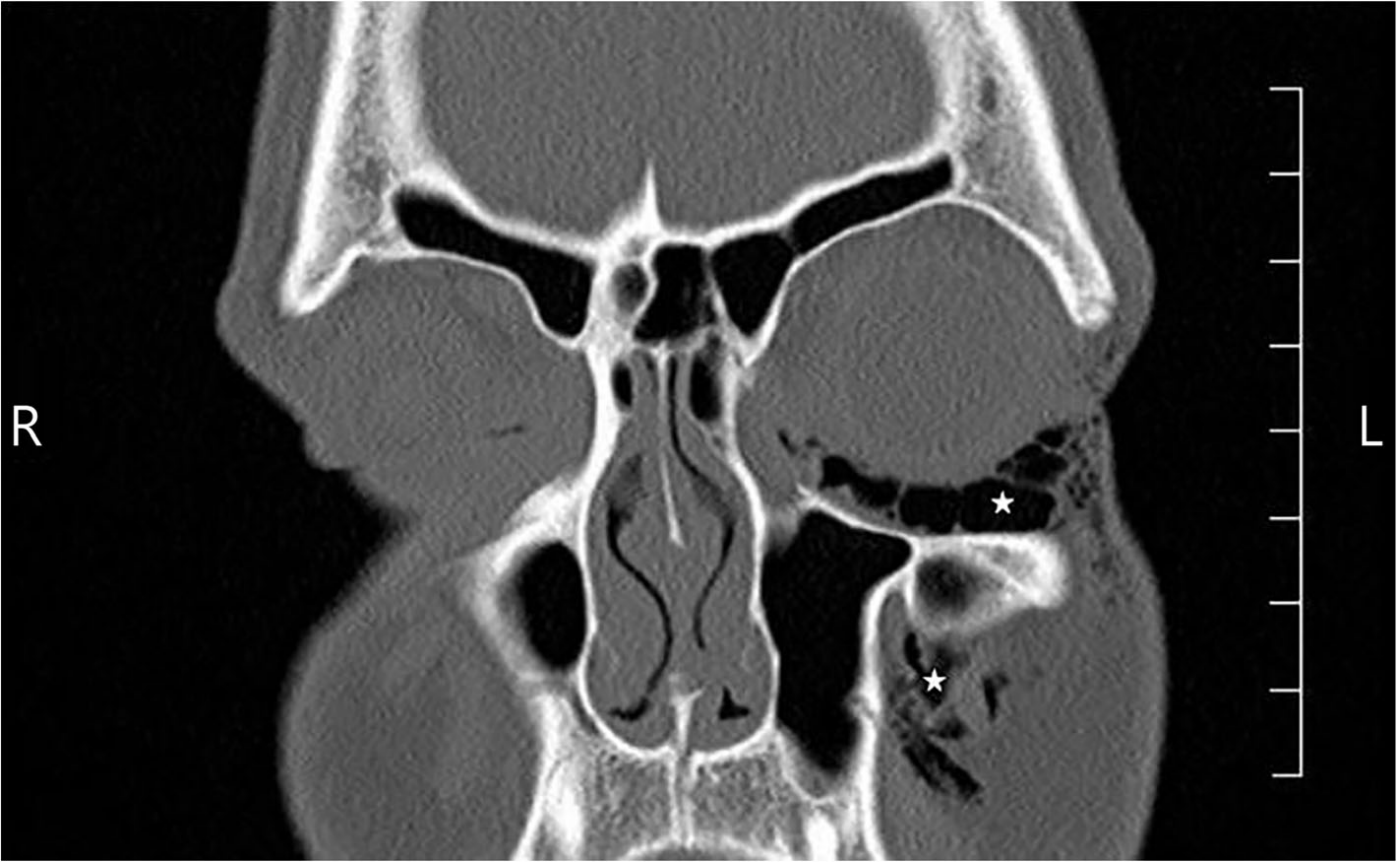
Coronal noncontrast CT of the orbits. Coronal CT of the orbits demonstrates the extent of subcutaneous emphysema within the intraorbital and periorbital soft tissues (starred)
